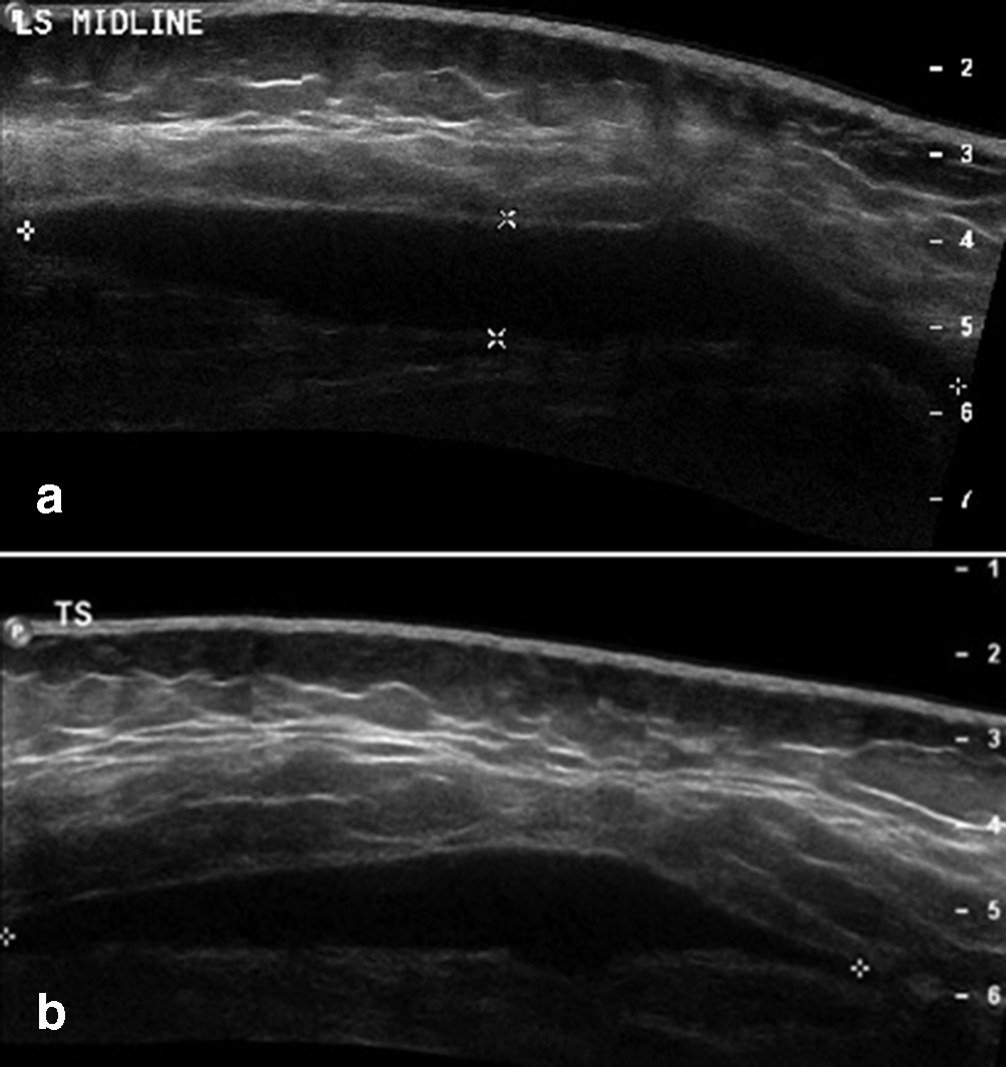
(a) Longitudinal and (b) transverse initial ultrasound of retrosacral Morel-Lavallée lesion at presentation, prior to aspiration. There is a hypoechoic focal fluid collection lying between the deep margin of the subcutaneous fat and the underlying fascia.
